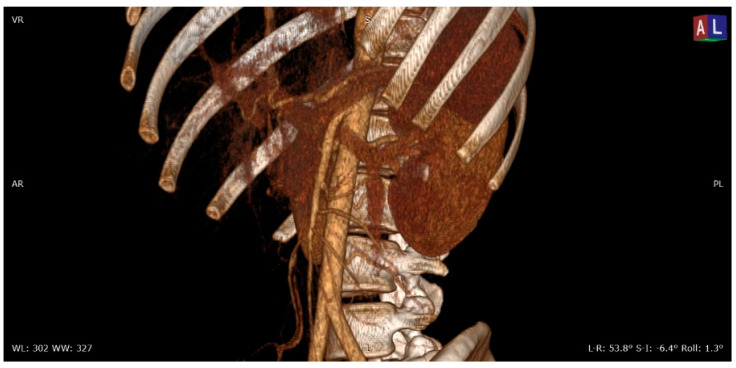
3D volume rendering (VR) contrast enhanced CT scan (portal venous phase) shows the relationships between the aorta, the superior mesenteric artery, and the left renal vein in a patient with nutcracker syndrome; in this acquisition, an initial enhancement of the left gonadal vein is also appreciable, which appears dilated (varicocele).
